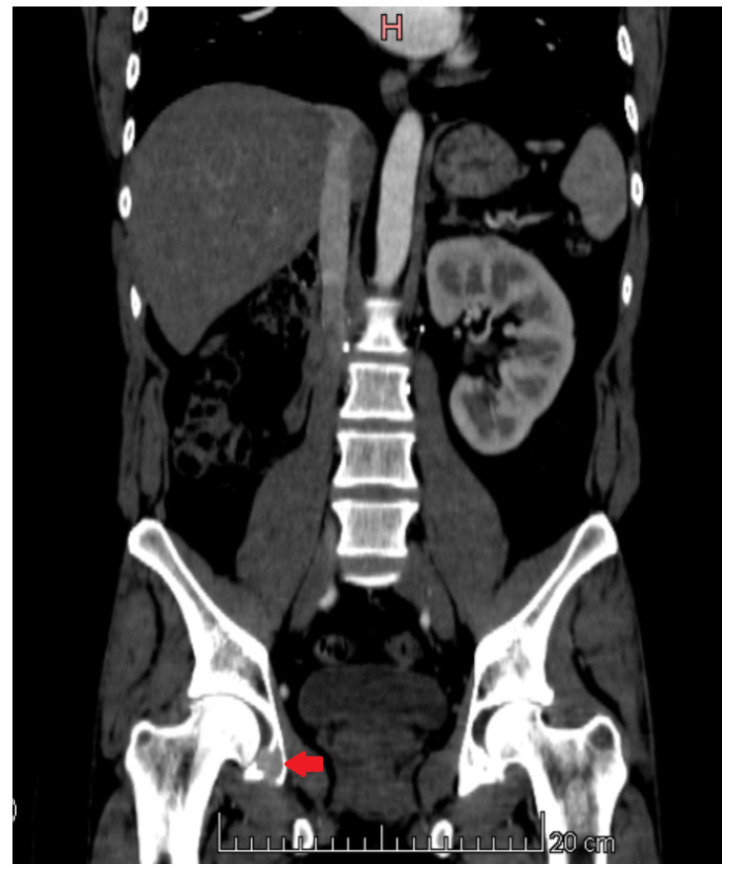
CT image. Osteoclastic-type metastatic tumour in the pelvic bones.
